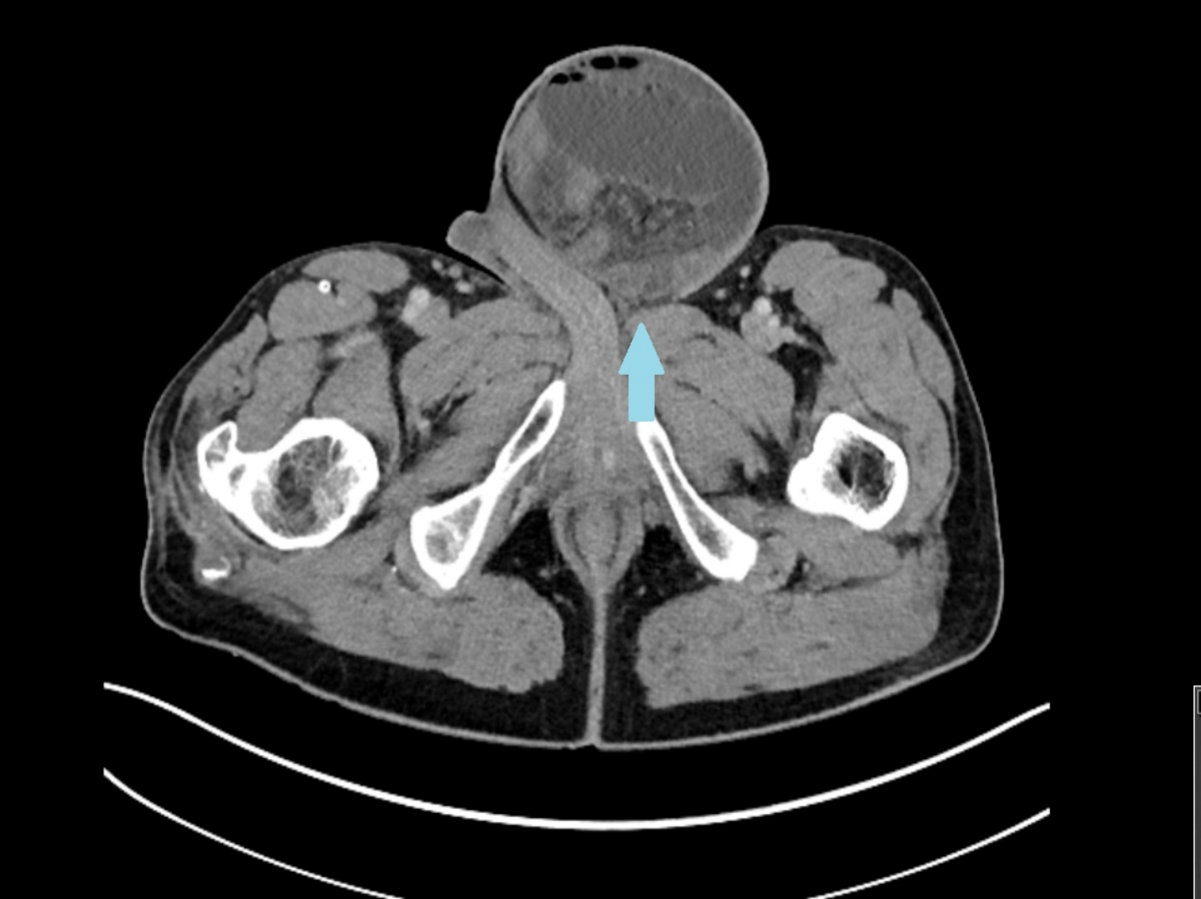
Abdominal computed tomography, axial plane Left inguinal hernia with narrow neck (arrow) contains dilated small bowel loops. Infiltration of fat, engorgement of herniated vessels and fluid are also noted.
